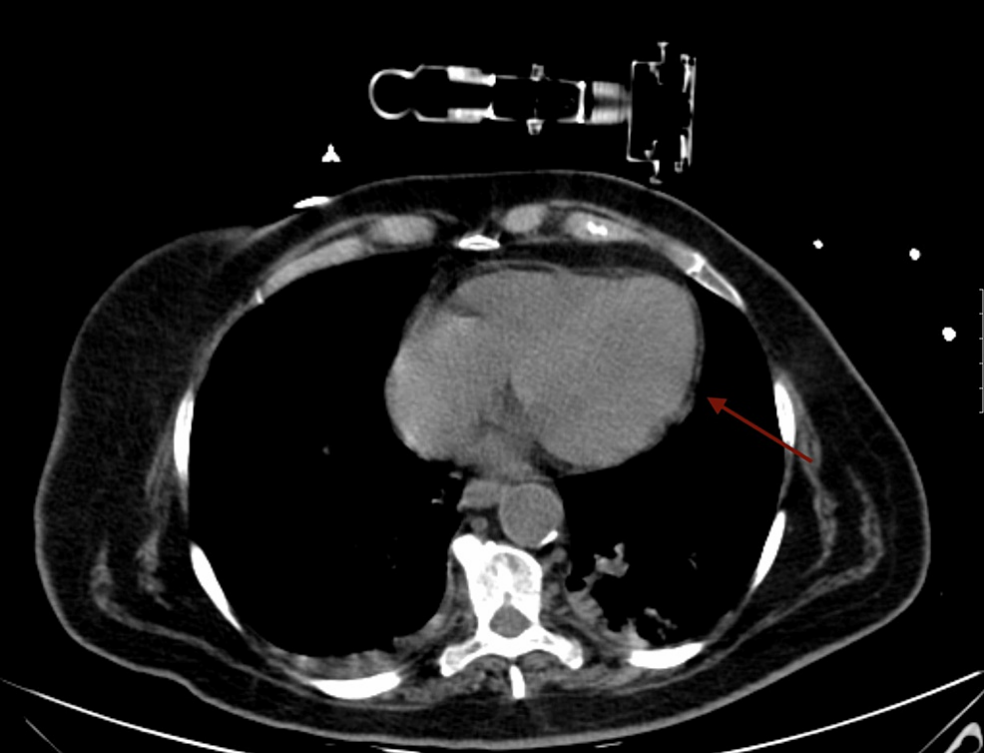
Control CT Control CT revealed the absence of pericardial effusion (arrow) and pneumomediastinum CT: computed tomography
